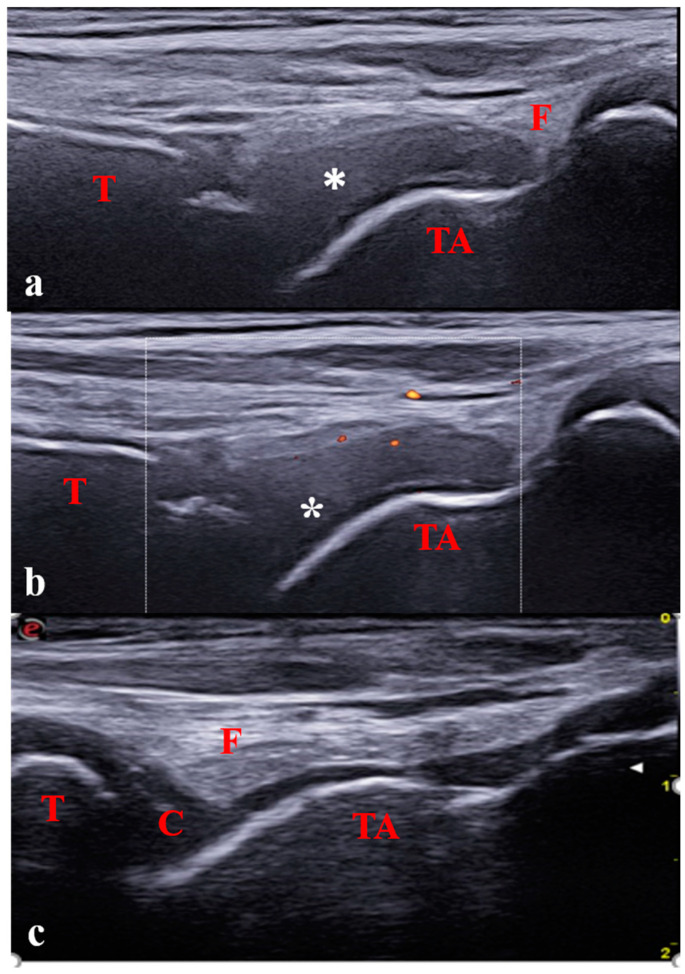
Longitudinal anterior scan of the tibiotalar joint in a 7-year-old with oligoarticular JIA; (a,b) Severe synovitis (B-mode grade 3) with minimal Doppler activity (grade 1). (c) Physiological aspect of the contralateral joint. C: cartilage; T: tibia; TA: talus; F: fat; * Synovitis.
